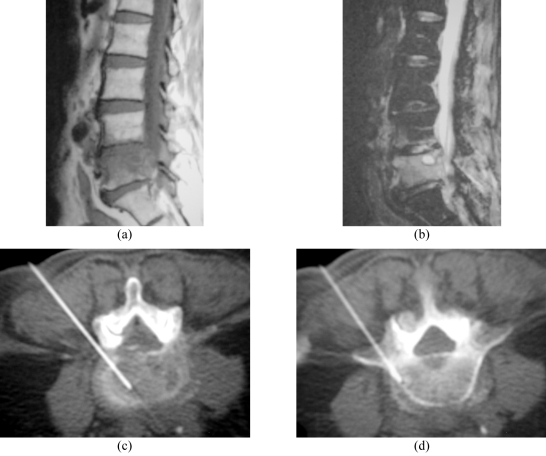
L4/5 infective spondylodiscitis in a 64-year-old man. (a) Sagittal SE T1-W MR image shows an ill-defined hypointense lesion involving most of the L5 vertebral body, with irregularity of the superior endplate. (b) Sagittal fat-suppressed FSE T2-W MR image shows hyperintense signal in the L5 vertebral body and the L4/5 disc, with anterior subligamentous and posterior extradural extension. Appearances are typical of L5 vertebral osteomyelitis with L4/5 discitis. Axial CT fluoroscopic images taken with the patient lying prone show the Ostycut needle inserted into the (c) L4/5 disc and (d) L5 superior subchondral vertebral body via a left posterolateral paravertebral approach.
